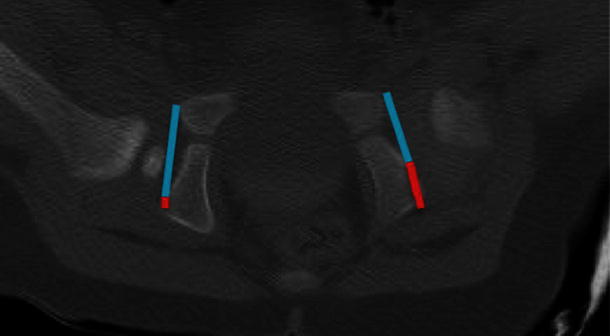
Postreduction single slice CT image highlighting the acetabular ratio
